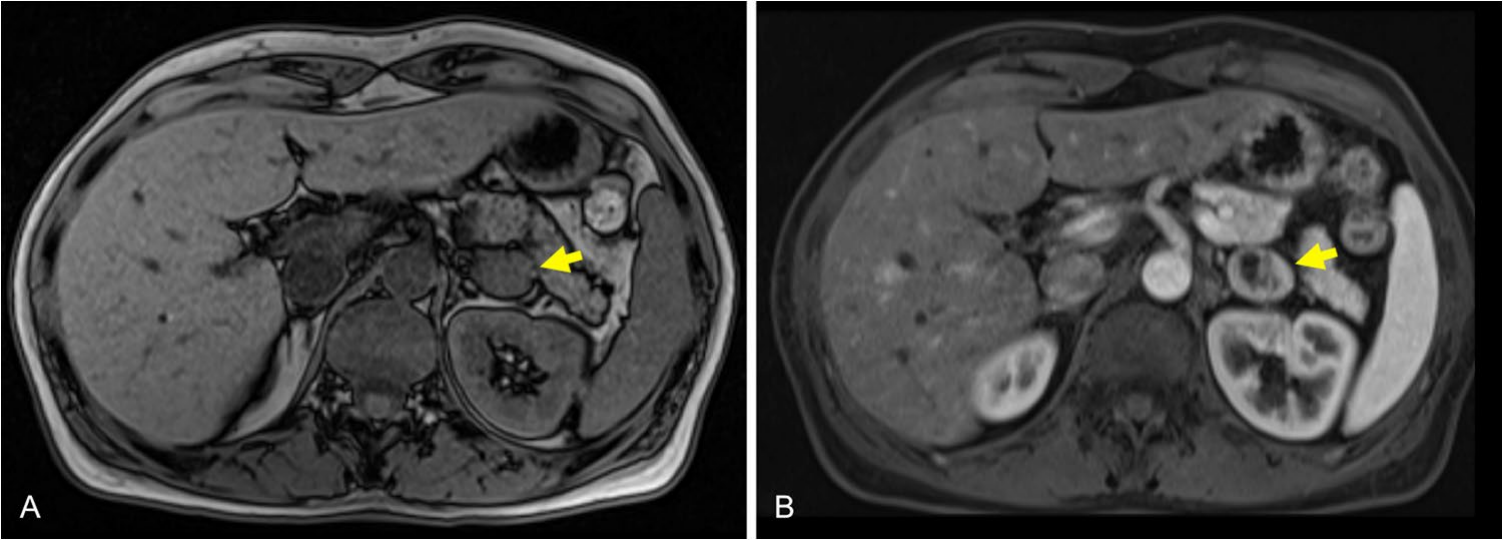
Magnetic resonance images demonstrate a left adrenal mass (arrows) with solid and cystic components. The solid component shows no significant loss of signal intensity in T1-w out-of phase sequence, which meanss that the lesion does not contain a significant amount of intracellular lipids (A). The arterial phase demonstrates dishomogeneous enhancement of the lesion and a lack of enhancement of the cystic component, a finding more frequently observed in pheocromocytoma (B). The diferential diagnosis includes lipid poor adenoma and pheocromocytoma
